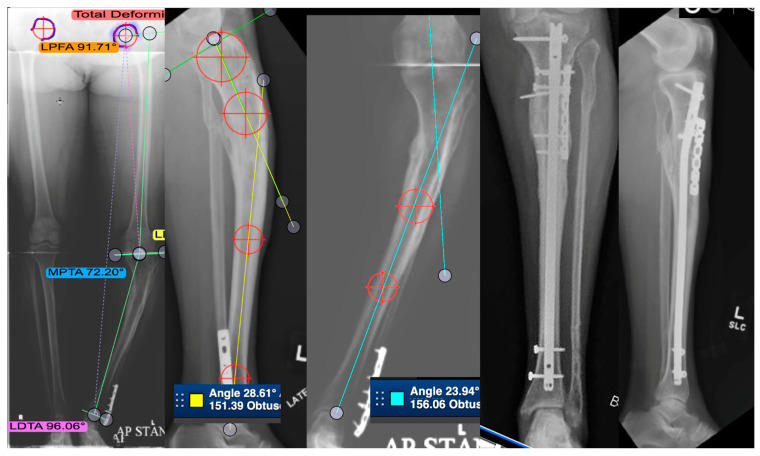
This is a case of a 53-year-old patient with post-traumatic tibial deformity complaining of knee and ankle pain. The deformity was assessed on long-leg standing alignment views, compared to the other tibia in AP and lateral views. CT scan revealed very little rotational deformity. An osteotomy was planned at the center of rotation and angulation (CORA), which was close to the same location on both AP and lateral. This is a single-plane deformity, which has been measured on both the AP and lateral images. The right two images show the patient post-transverse opening-wedge osteotomy fixed with an intramedullary nail and a plate. Healed in good alignment.
